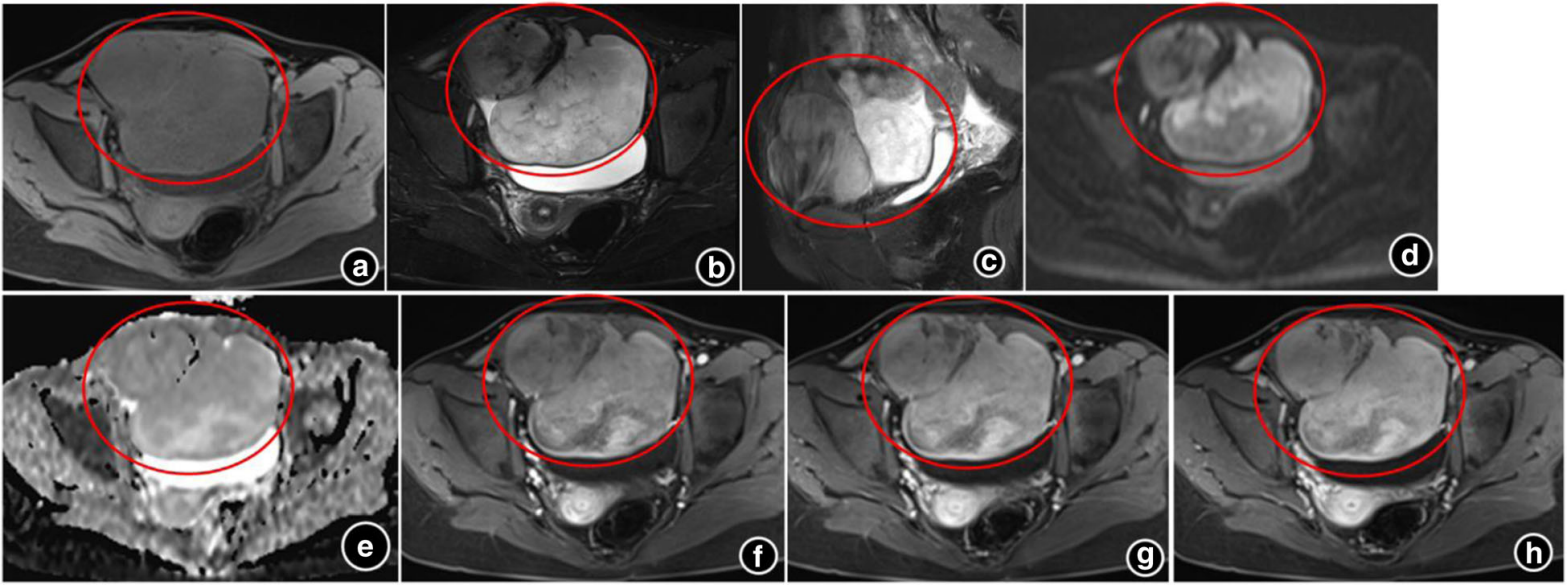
The magnetic resonance imaging findings of low grade fibromyxoid sarcoma. a: T1-weighted MR images; b: T2-weighted MR images; c: Sagittal T2WI showed that the rectus abdominis was open with umbrella shape; d: The tumor showed high signal intensity on diffusion weighted imaging; e: The tumor showed low signal intensity on apparent diffusion coefficient (ADC) map; f-h: On Gadolinium-enhanced T1-weighted MR images with fat suppression at arterial (f), portal (g) and delayed phases (h), the tumor showed heterogeneous enhancement
